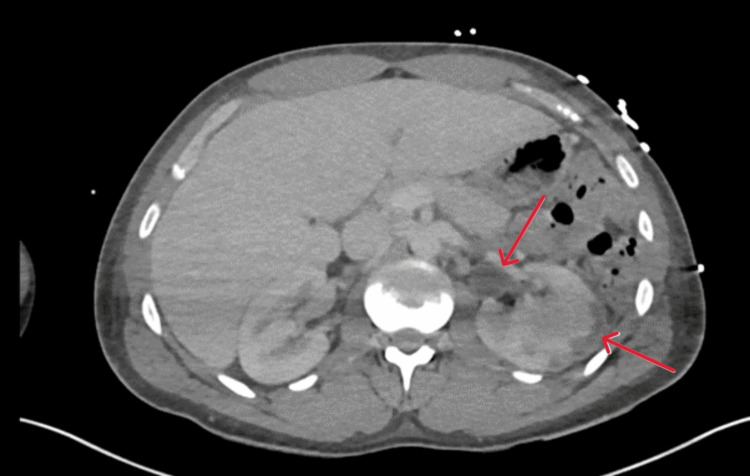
A CT abdomen on Day 5 showed worsening appearances of the left-sided severe pyelonephritis and lobar nephronia with a subcapsular collection measuring up to 1.2 cm in depth. There is mild hydronephrosis and hydroureter with an enhancing lumen, consistent with ongoing inflammation. The red arrows show left-sided severe pyelonephritis and lobar nephronia.
